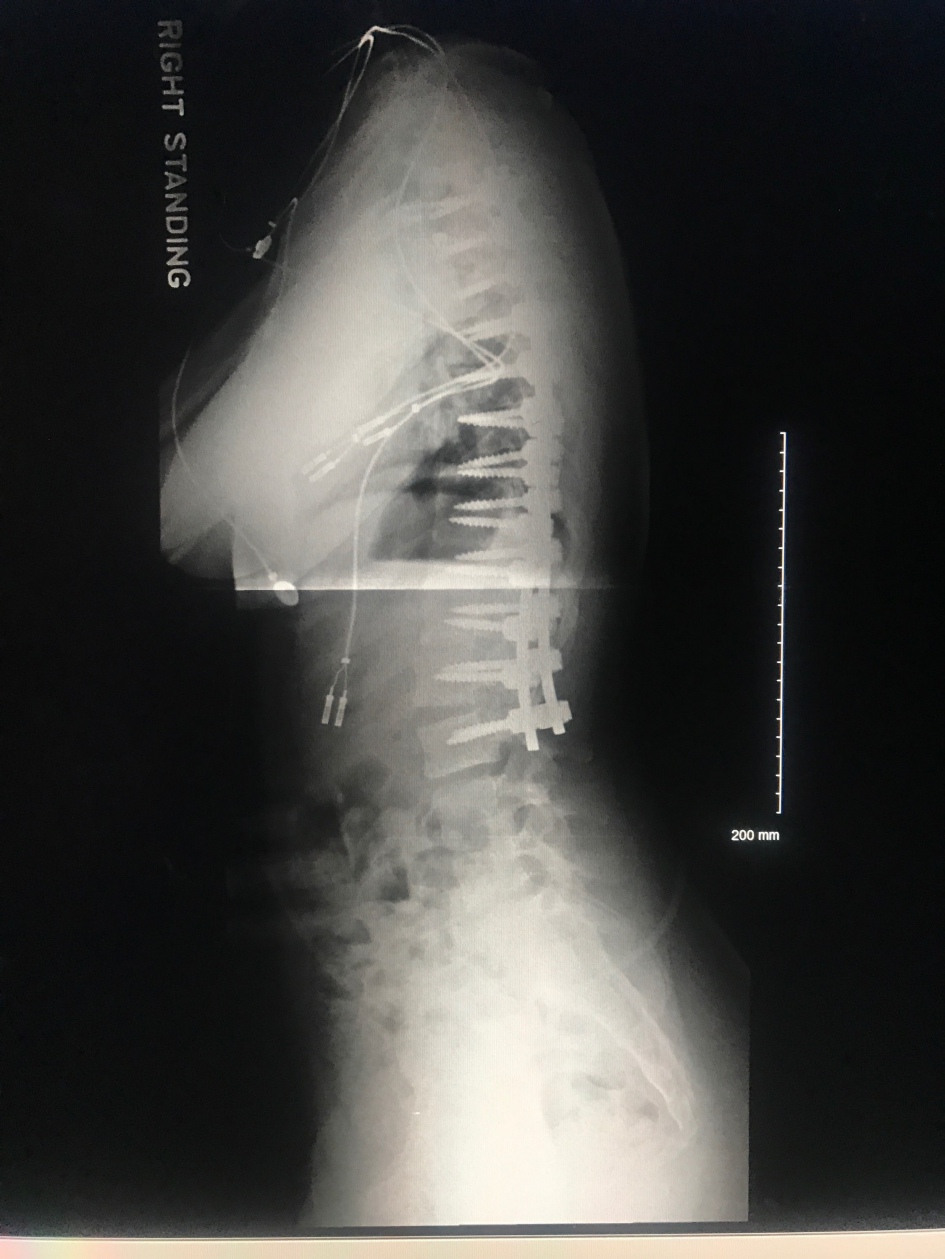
Postoperative lateral view of 36-inch standing scoliosis radiograph.
